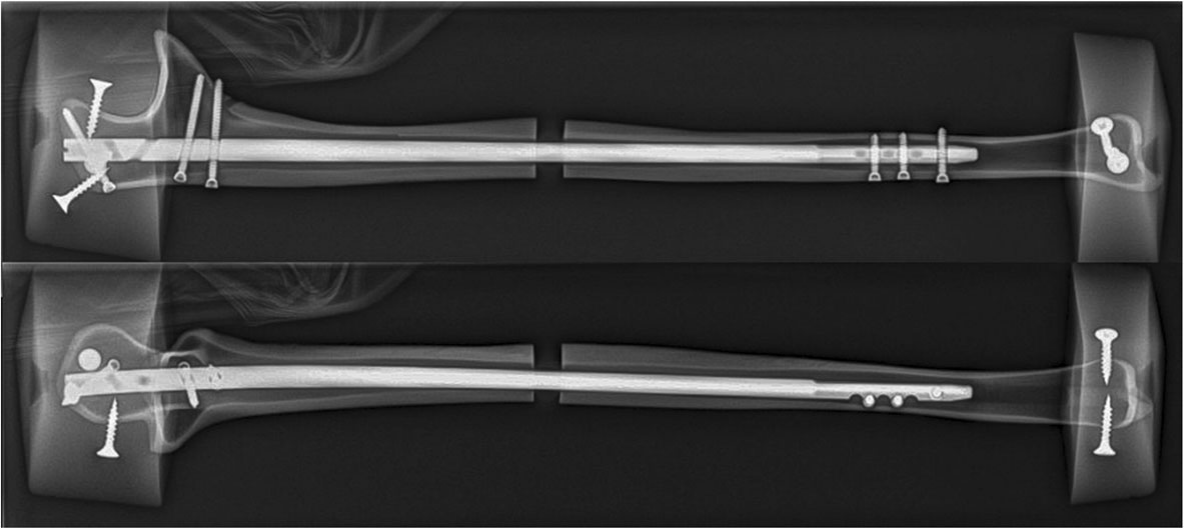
X-ray in anterior-posterior and lateral view of a left nail with inserted locking screws after potting in PMMA cement
